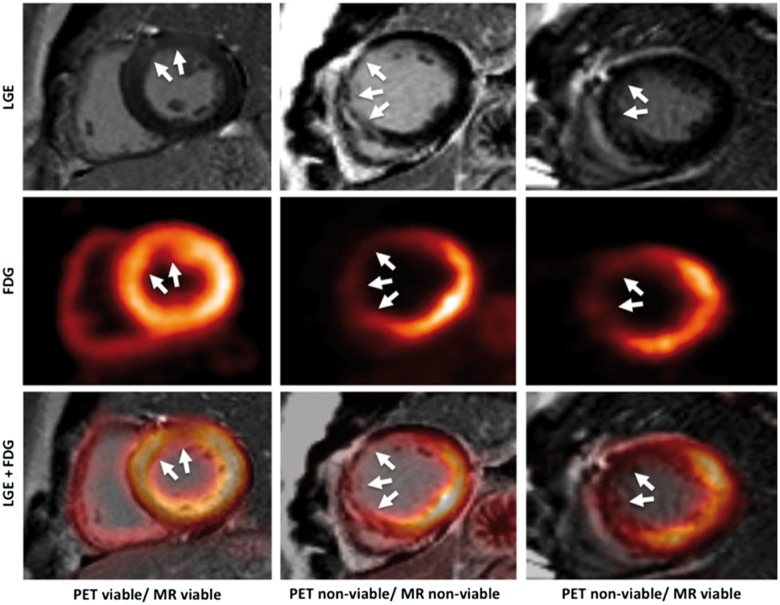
Different combinations of ^18^F-FDG uptake and LGE transmurality with PET-MR. Left column: ‘PET viable/MRI viable’; middle column: ‘PET non-viable/MRI non-viable’; right column: ‘PET non-viable/MRI viable’. White arrows indicate infarcted myocardium. Reproduced with permission from ref. 44.
